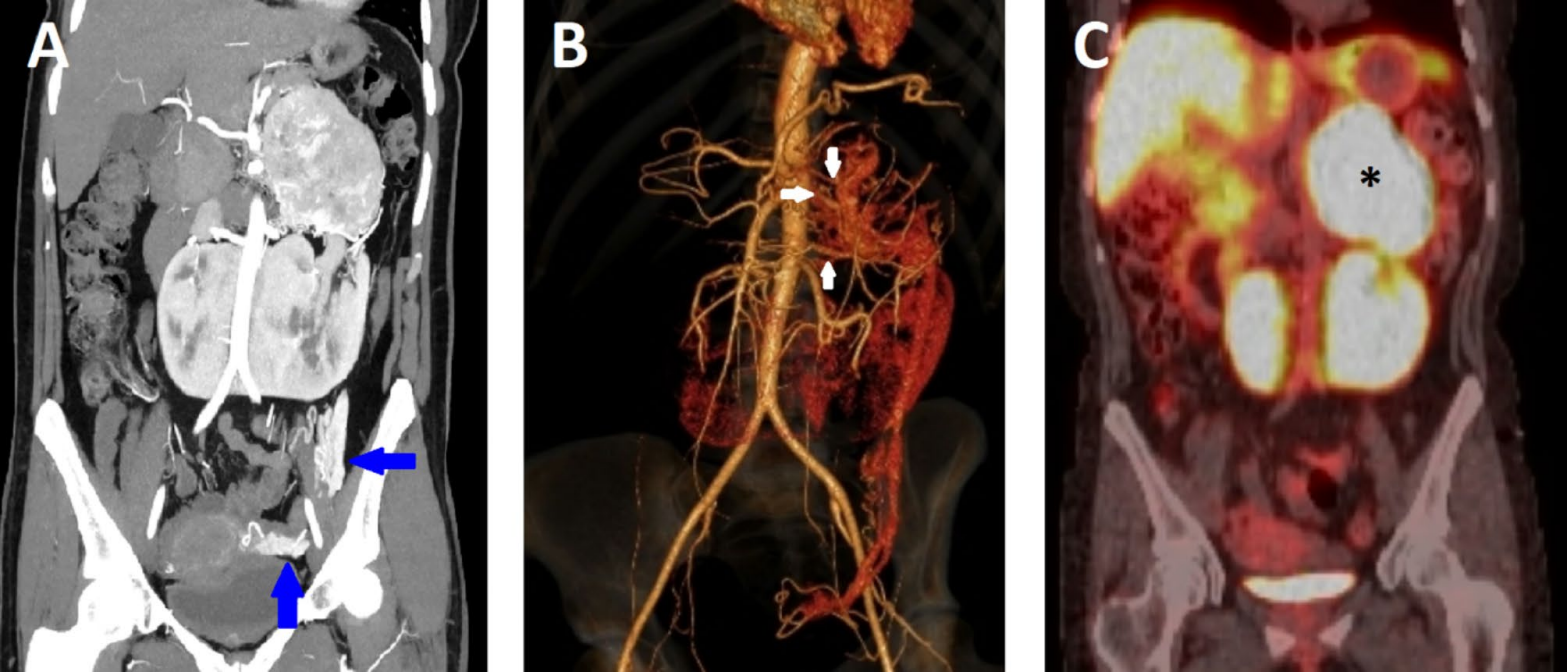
(A.) The preoperative CT angiogram, Maximum Intensity Projection (MIP), in the coronal plane shows a dilated left ovarian vein and uterine plexus (blue arrows) suggesting congestion caused by blockage of retro-aortic left renal vein outflow due to the large adrenal mass and aortic compression. (B.) The 3D vascular reconstruction demonstrating arterial vascular supply of the mass (white arrows). (C.) PET/CT Copper Cu 64 Dotatate demonstrating the large intensely Dotatate avid mass (black asterisk) superior to the left moiety of the patient’s horseshoe kidney and no evidence of Dotatate avid metastatic disease
